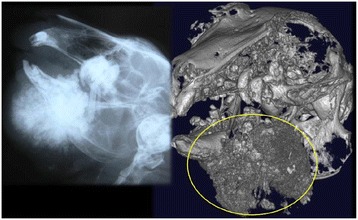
Comparison between the radiologic findings and a CT image of lower jaw osteolysis. Radiographic image of mandibular osteolysis; inset, the CT image.
